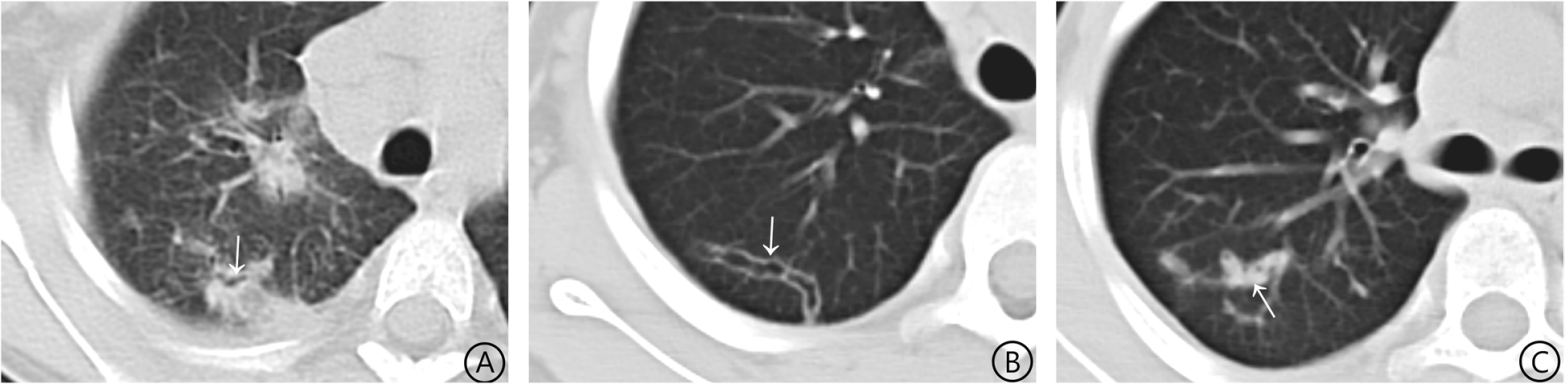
Figure 1 On CT, there are pulmonary tunnel signs in patients with paragonimiasis. A Multiple patchy consolidation shadows in both lungs, with tunnel signs (white arrow) within the consolidation shadows in the right upper lobe; B The consolidation surrounding the tunnel shadow of the right lung's upper lobe has been absorbed, leaving only a low-density tunnel shadow (white arrow); C Iso-density shadow completing the tunnel shadow of the right lung's upper lobe (white arrow)
